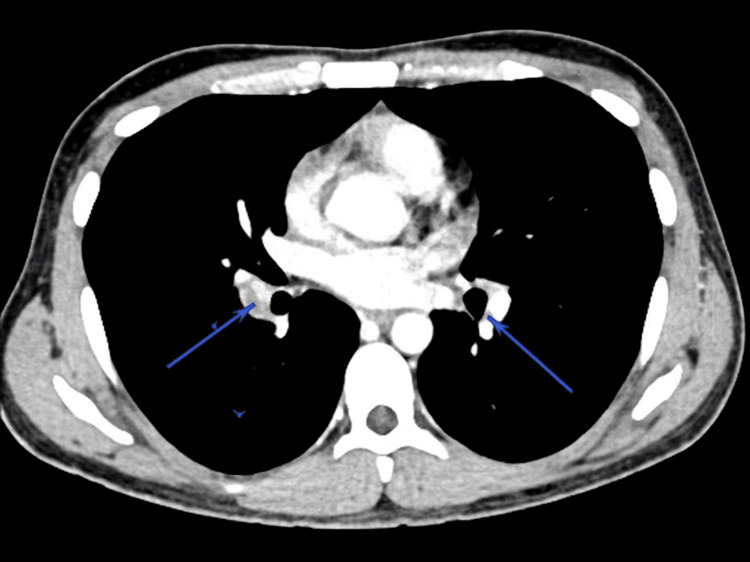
Contrast CT chest showing bilateral pulmonary emboli (PE) Arrows pointing to filling defects consistent with PE diagnosis
